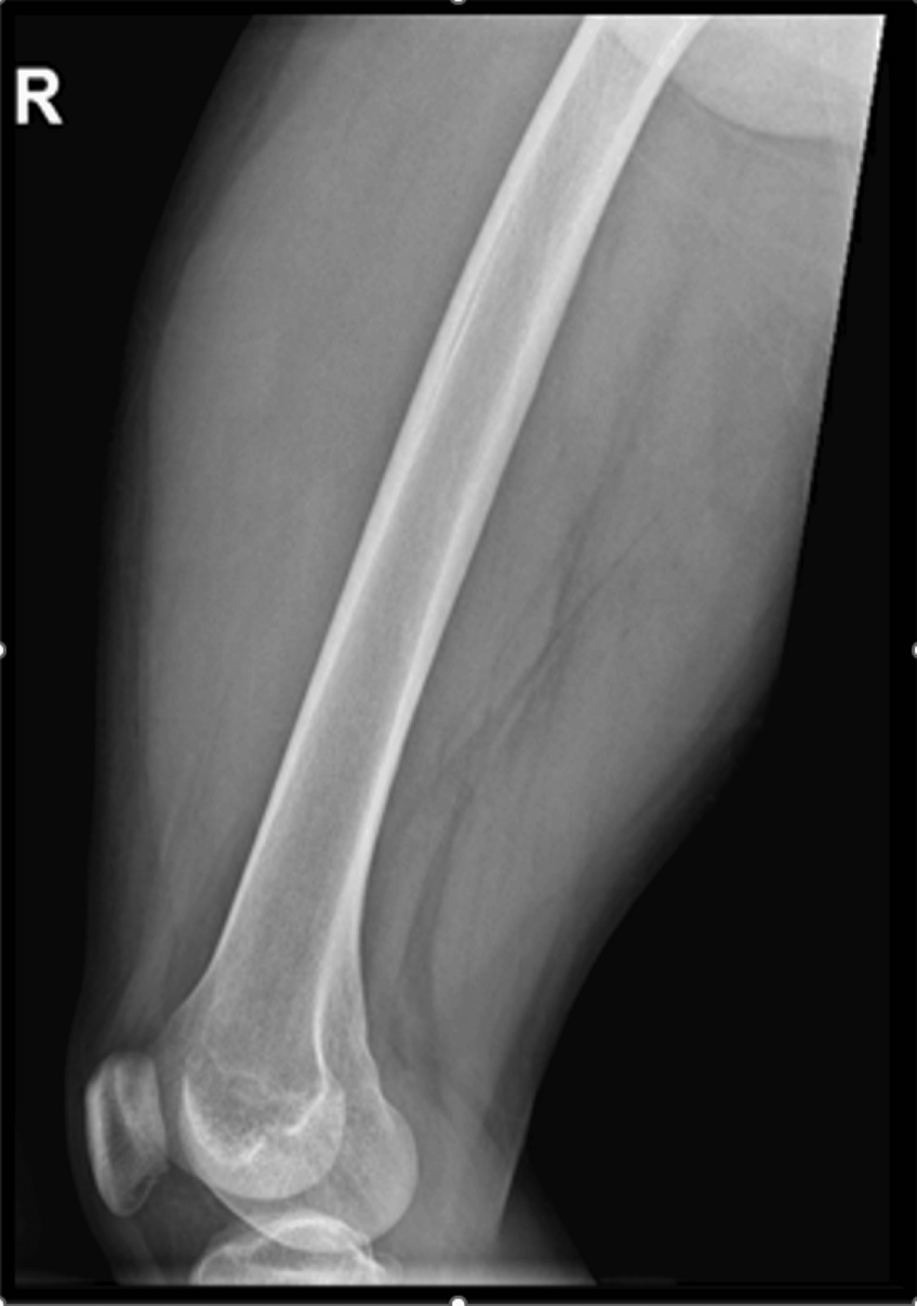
Lateral X-ray view Normal X-ray of the femur bone
